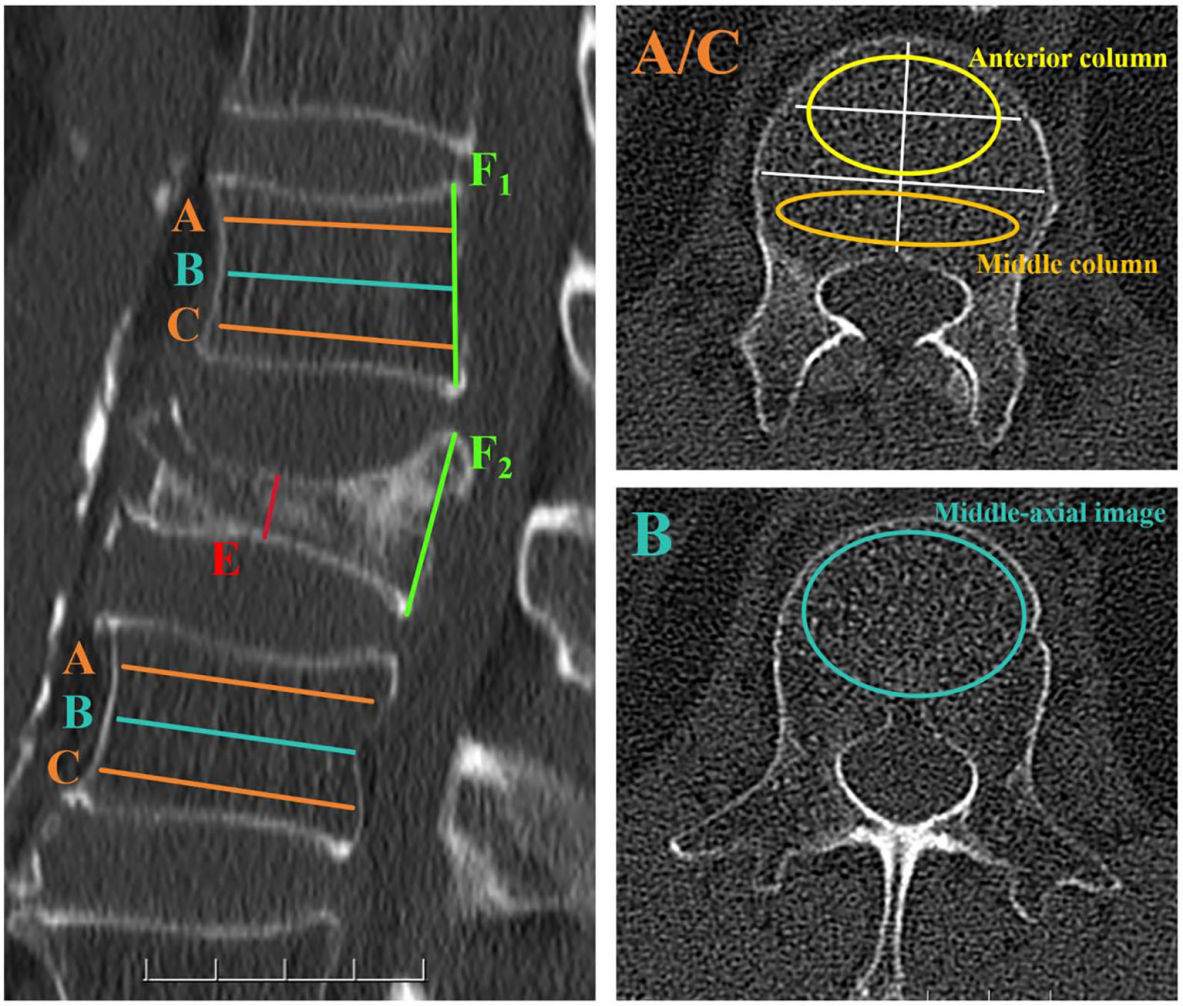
Computed tomography (CT) scan demonstrating the method for determining the HU value using an elliptical ROI. In the left - hand image, the axial slices of interest are shown on a sagittal slice of a CT scan of the lumbar vertebra. Slice A was obtained inferior to the superior end plate, while slice C was taken superior to the inferior end plate. Slice B represented the middle - axial image of the vertebral body. For slices A and C, two distinct locations on each axial slice were selected as ROIs for HU measurements: the anterior two - thirds of the vertebrae and the posterior one - third of the vertebrae. For slice B, a large elliptical area was used as an ROI to measure HU values. Elliptical ROIs were drawn as large as possible, excluding cortical edges to prevent volume averaging. The compression ratio of osteoporotic vertebral compression fractures was determined by E/F1. If no compression occurred at the posterior edge of the fractured vertebra, E/F2 was used to determine the compression ratio.
